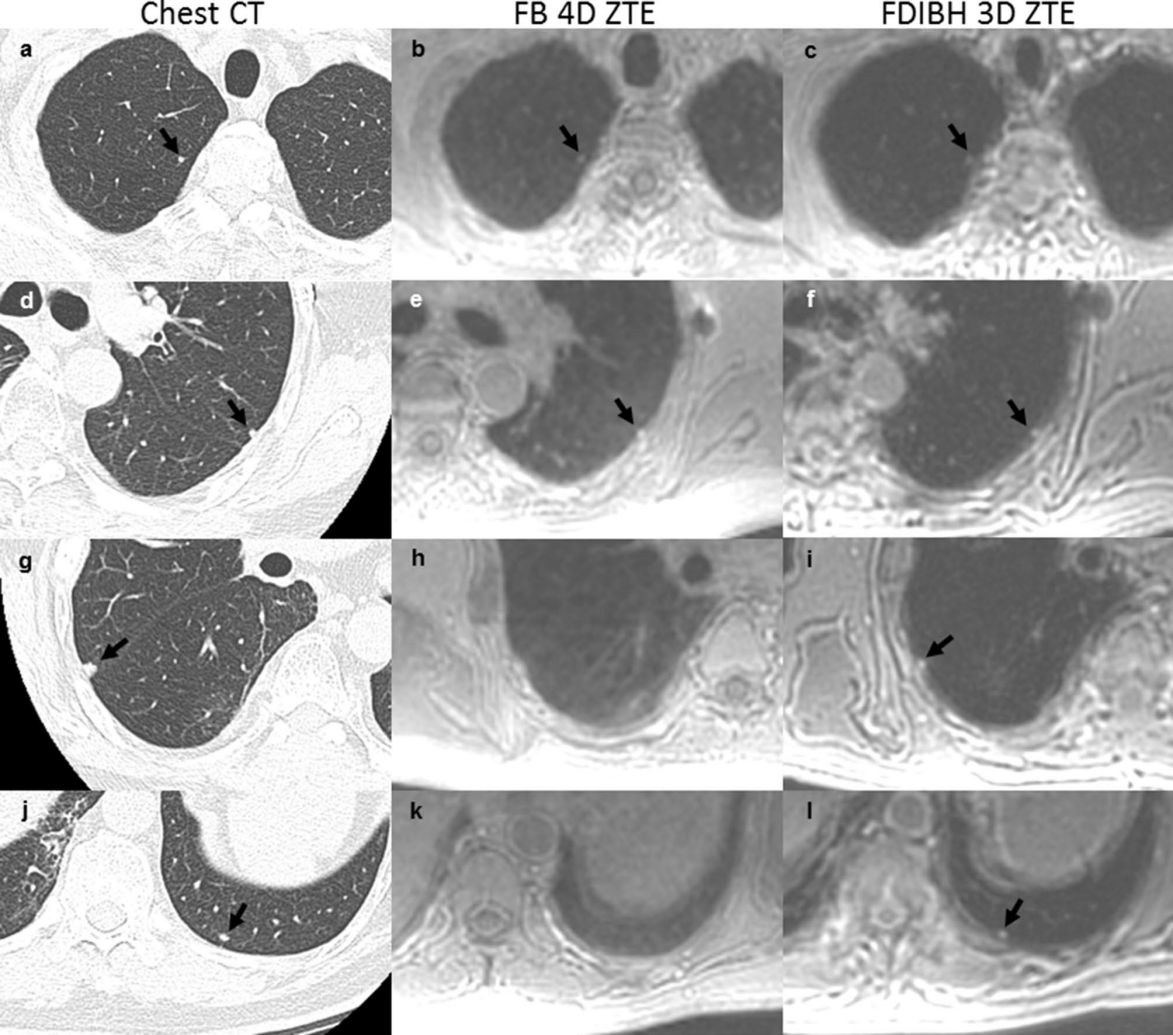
The traditional dual-echo gradient T1-weighted images and the FDG images failed to detected lung metastases in a 63-year-old male thyroid cancer patient. The FB 4D ZTE (b, e) and the FDIBH 3D ZTE (c, f) detected a 2.9 mm right upper lobe nodule and a 3 mm left upper lobe nodule. The FDIBH 3D ZTE additionally detected a 5 mm right upper lobe nodule (i) and another 4 mm left lower lobe nodule (l). All the detected nodules were identified by arrows. The lung volume of the FDIBH 3D ZTE was more similar to that of the full expansion chest CT than that of the FB 4D ZTE.
